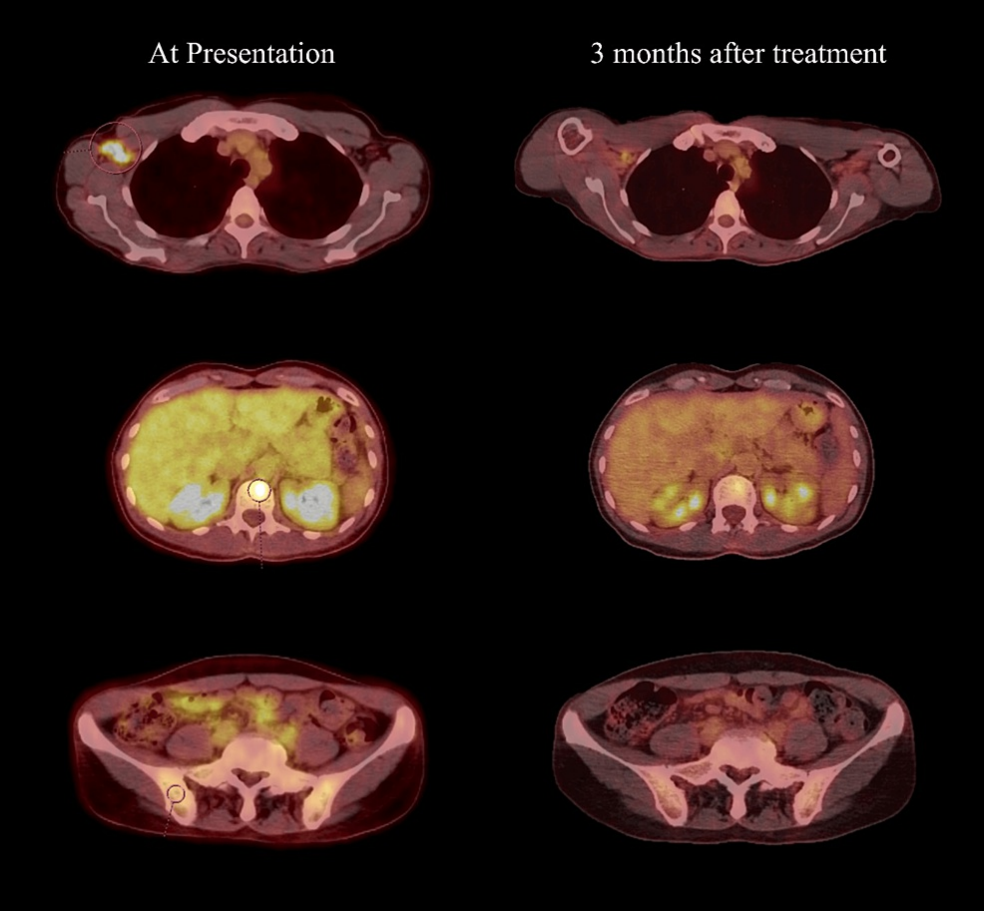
PET scan of patient PET scan at presentation (left column) showing FDG avidity in the left axilla (top), axial skeleton (middle), and right iliac wing (bottom) with a significant decrease in all sites after treatment initiation (right column). Areas of avidity noted by circles in each image at presentation. PET, positron emission tomography; FDG, fluorodeoxyglucose
